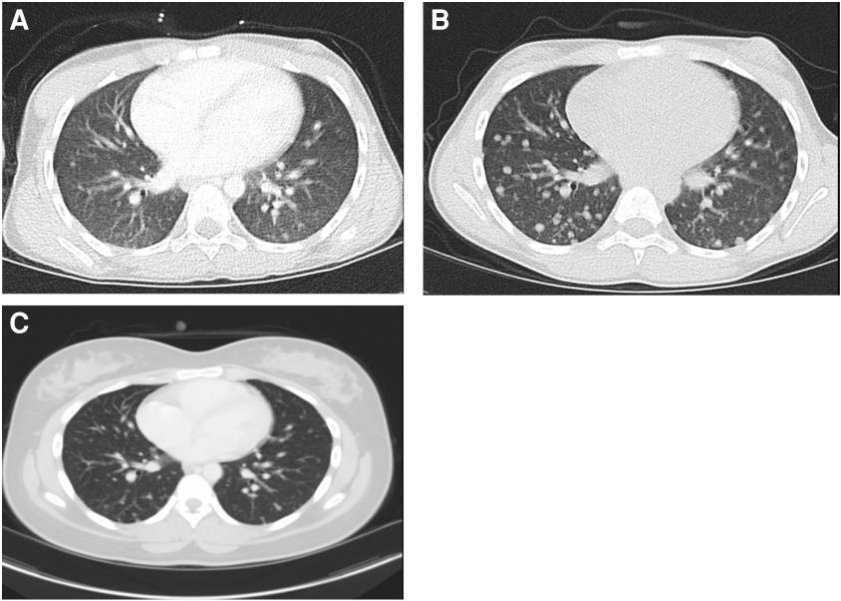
Computed tomography (CT) of the chest. (A). January 2016: initial CT of the chest demonstrating diffuse pulmonary nodules. (B). May 2017: follow-up CT of the chest with increased size and number of pulmonary nodules 6 months after second RAI treatment. (C). September 2023: follow-up CT of the chest with stable/unchanged pulmonary nodules.
